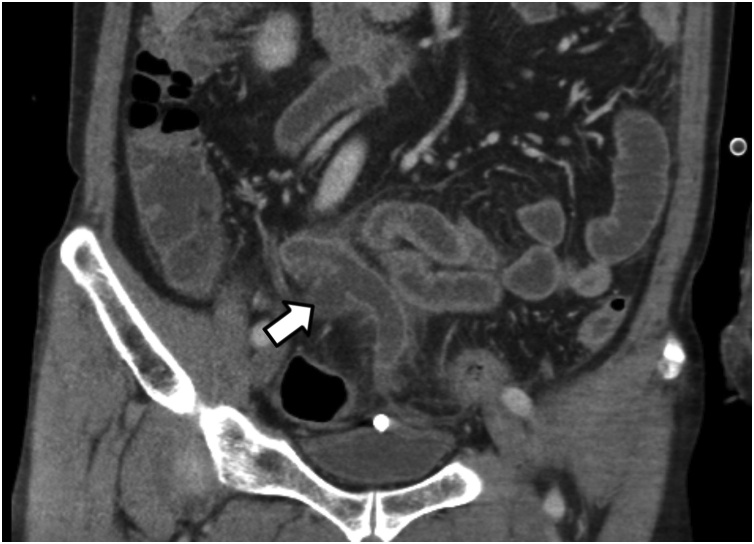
Preoperative CT imaging findings. An enlarged, perforated appendix with surrounding abscess indicated by a white arrow.
